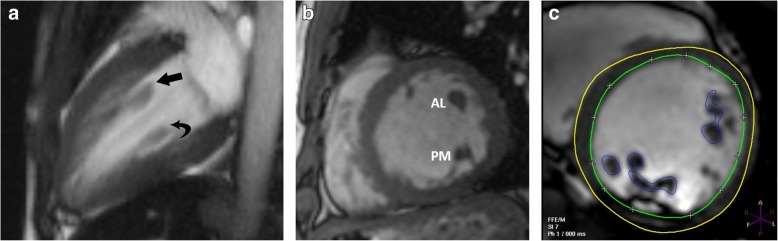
MRI appearances of normal papillary muscles. a Two-chamber vertical long-axis SSFP MRI through the left ventricle shows the anterolateral (straight arrow) and posteromedial (curved arrow) papillary muscles. b Short-axis SSFP MRI image shows the anterolateral (AL) and posteromedial (PM) papillary muscles. c Short-axis SSFP MRI image shows the technique for measuring papillary muscle mass. The papillary muscles have been contoured (blue) in end-diastolic image to derive papillary muscle mass. The endocardial (green) and epicardial (yellow) contours are also seen
